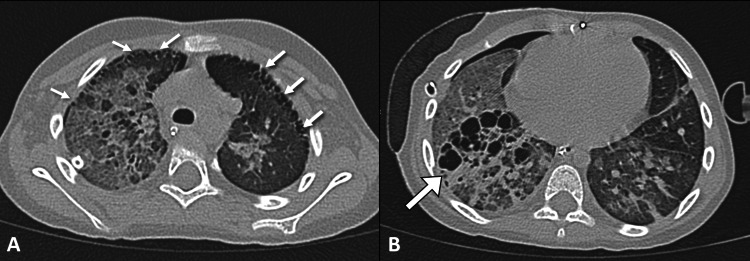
An axial CT scan of the chest at the level of the upper (A) and middle thorax (B) shows ground-glass opacity with interlobular septal thickening related to pulmonary edema along with subpleural cysts bilaterally (small arrows). The right middle lobe is significantly replaced by variable-sized cysts (long arrow). A small pneumothorax (related to chest tube insertion) is noted. CT: computed tomography
